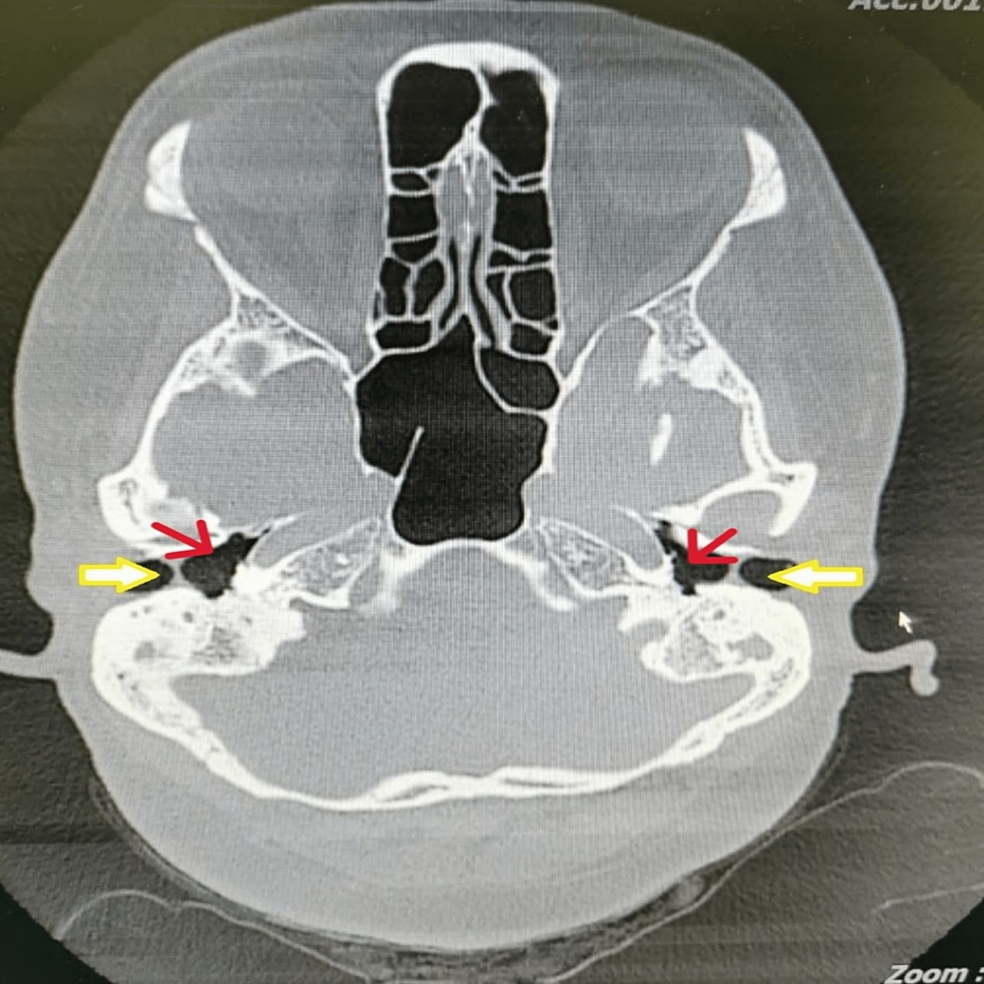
HRCT temporal bone shows crescentic soft tissue thickening (yellow arrow) overlying lateral to the tympanic membrane (red arrow) HRCT: high-resolution computed tomography
